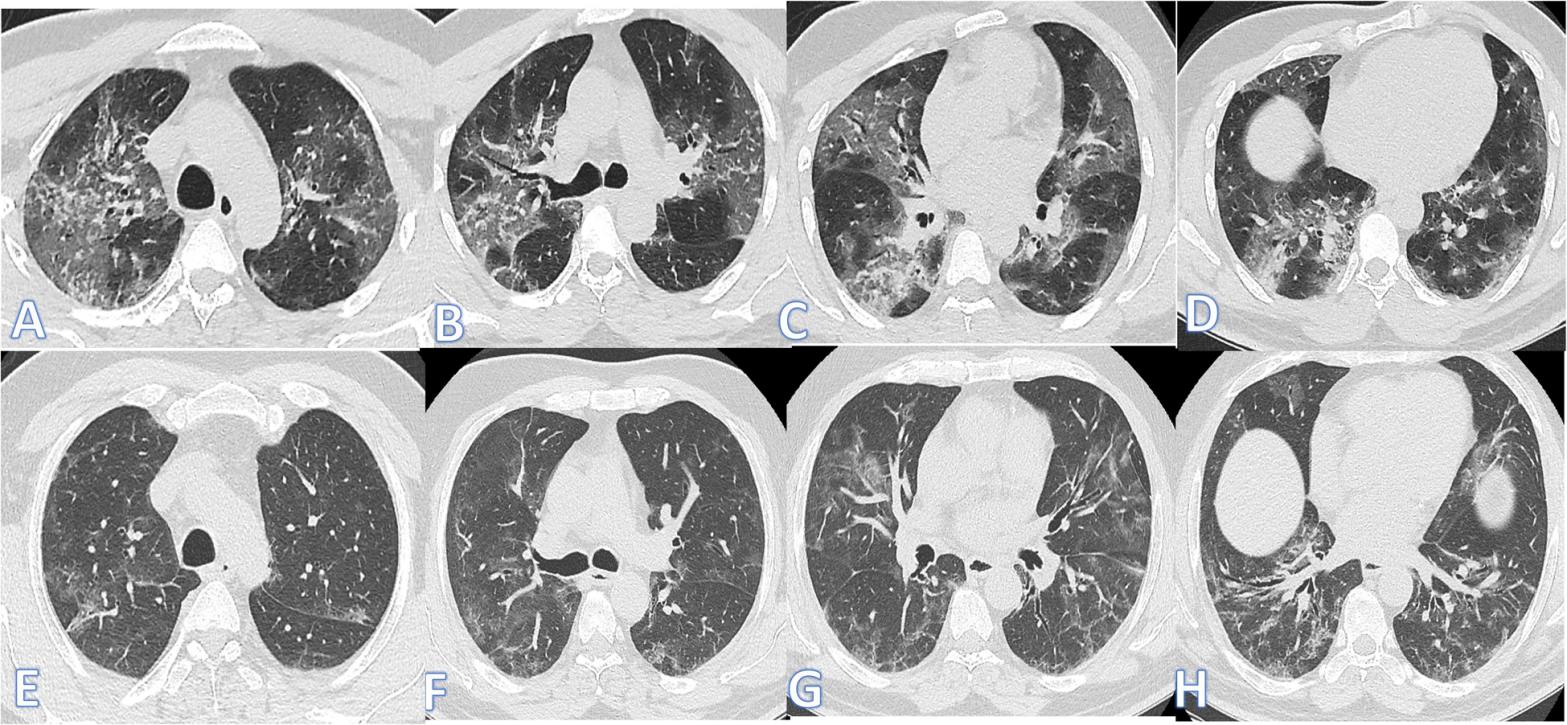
Thirty-nine-year-old male: he has DM and HTN; peak CT severity score 17; consolidation/crazy-paving score 13. He was admitted to the ICU; laboratory results showed lymphopenia, high CRP, d-dimer, and serum ferritin. Steroid was given; length of stay during hospitalization is 20 days. Peak CT during admission (a–d) showed bilateral GGO with mild interstitial thickening and basal consolidation patches. Follow-up CT 2months after discharge (e–h) showed lung fibrosis with parenchymal bands, coarse reticular pattern, and mild bilateral traction bronchiectasis
